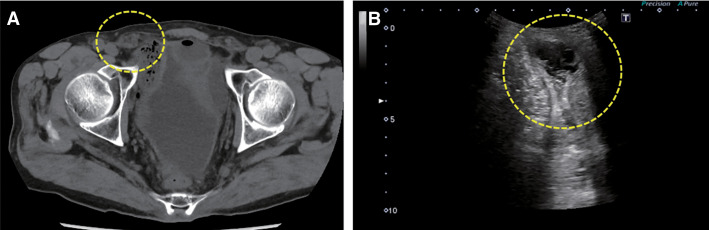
CT shows no hernia (A), but US shows an external inguinal hernia with small bowel prolapse in the right inguinal region (B). US, ultrasonography
